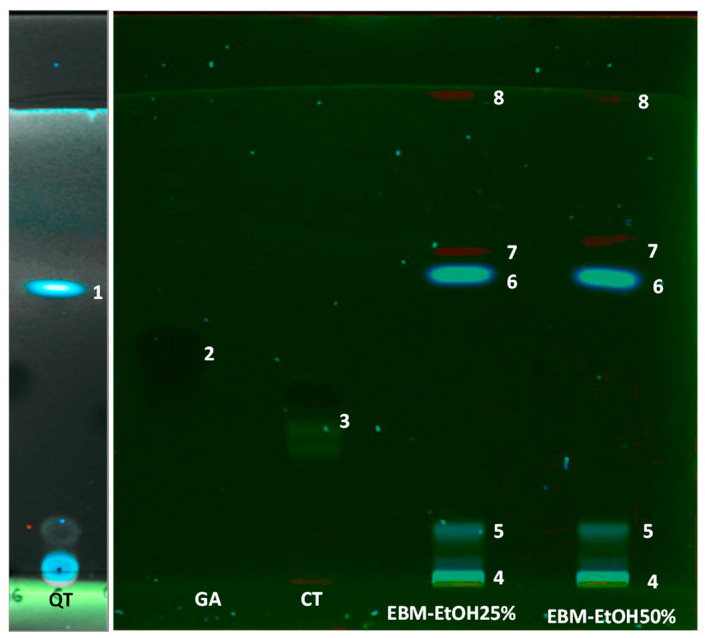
Analysis TLC of EBM-EtOH25% and EBM-EtOH50%. GA (gallic acid standard), CT (+ catechin standard) and QT (quercetin standard). UV light at 366 nm.
